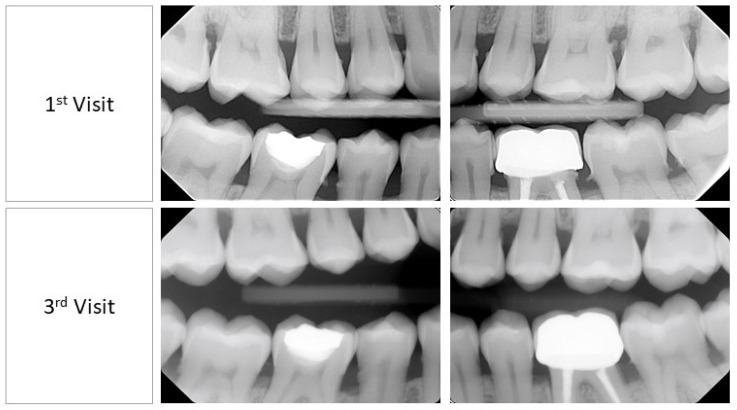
Bitewing radiographic examination at first visit: baseline and third visit: +14 days.
